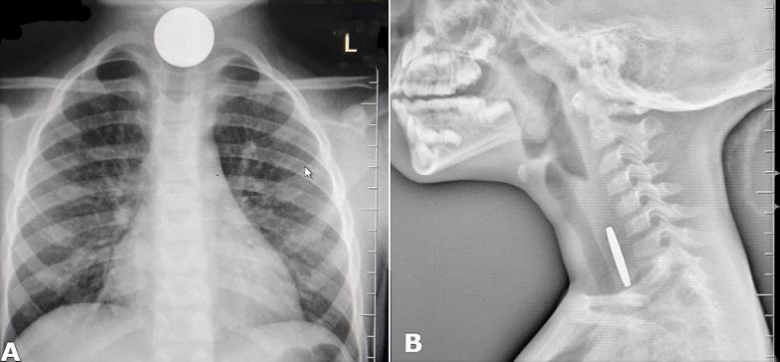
an impacted coin in the esophagus, AP (A), and Lateral (B) views of plain X-rays of the neck
